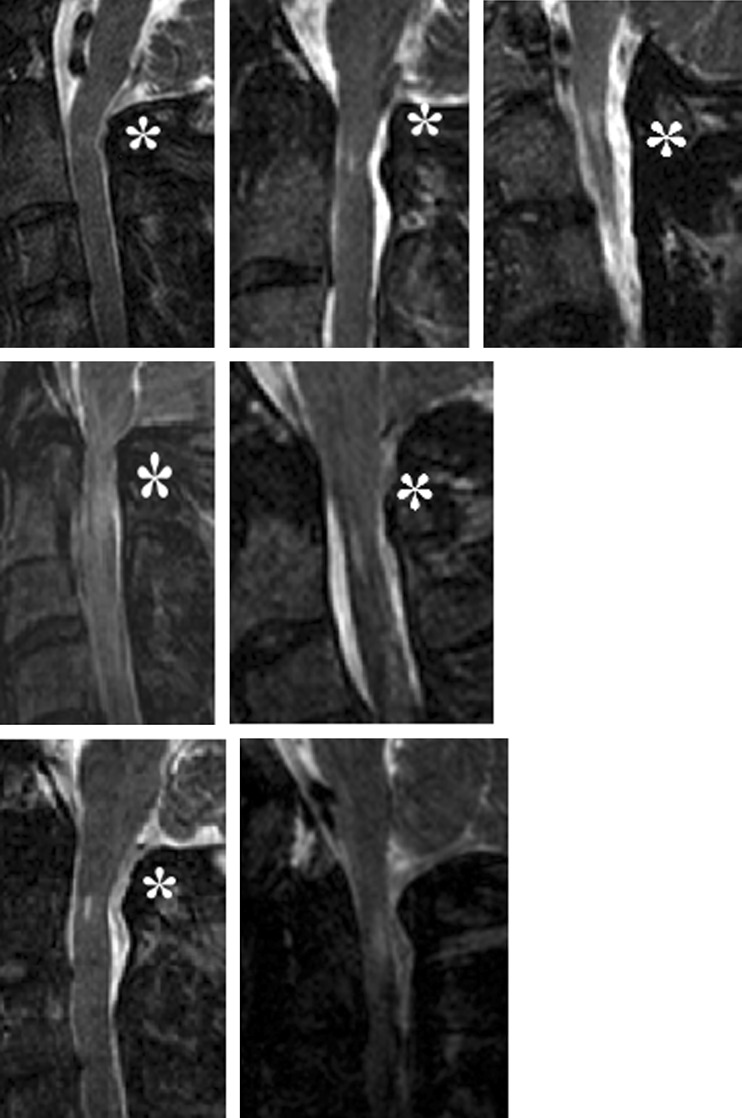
CHII lesions and thinning of the spinal cord. A CHII lesion was diagnosed when a typical high-intensity intramedullary signal was seen at the C0–C2 level on T2-weighted sagittal MR images in neutral position. All subjects demonstrating a CHII lesion are summarised here. Those subjects who demonstrated a CHII lesion and thinning of the spinal cord are marked with an *asterisk*
